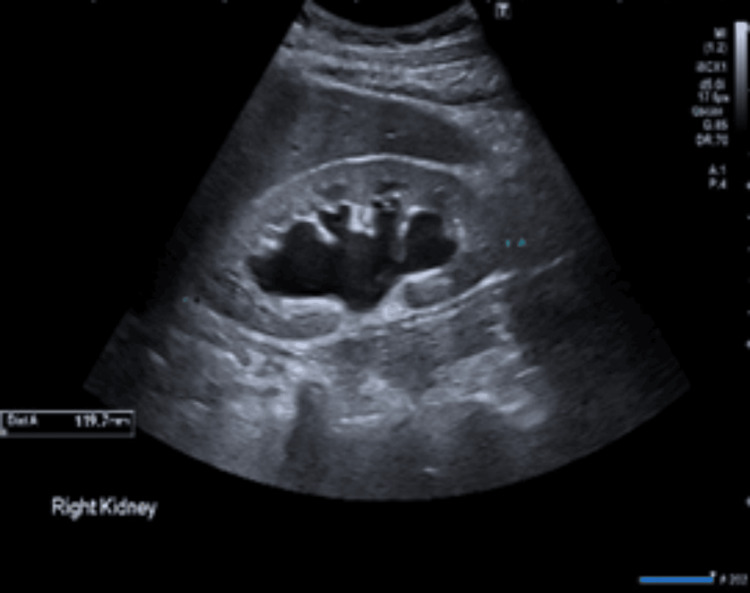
Ultrasound of the right kidney showing hydronephrosis.
